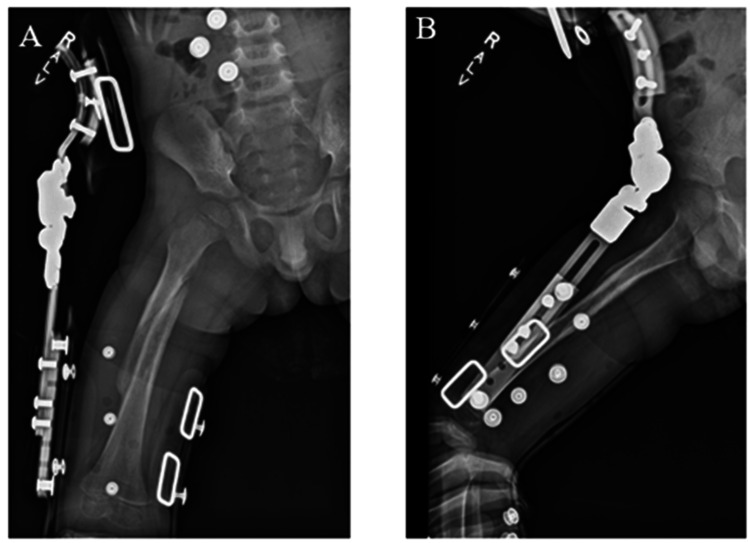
Final imaging for case 4, including (A) AP and (B) lateral radiographs of the right lower extremity demonstrating the healing of the spiral fracture of the femoral shaft
